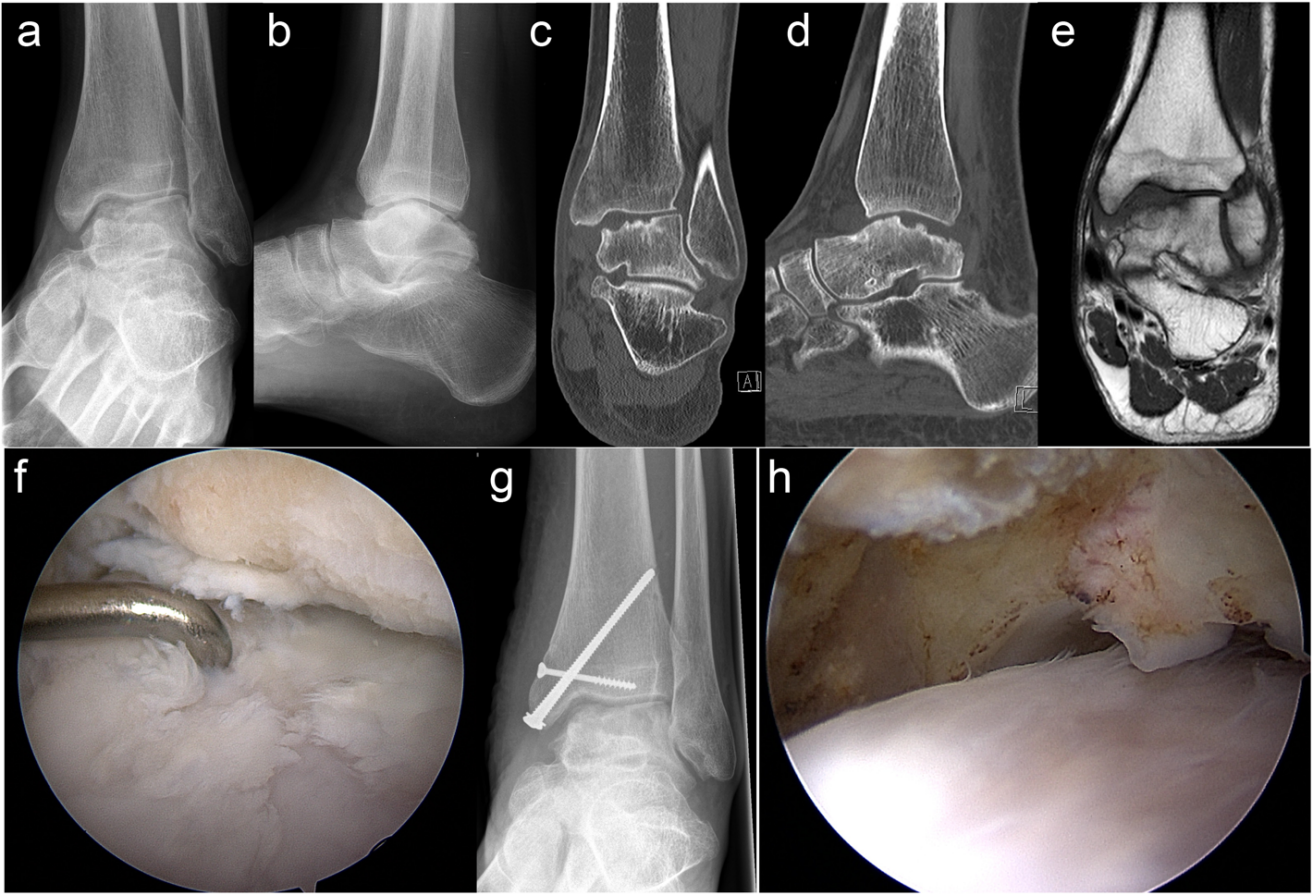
Eighteen-year-old male patient (case 11) with a posttraumatic osteochondral lesion of the medial talus, which was treated with primary combined matrix-associated autologous chondrocyte implantation (MACI) via medial malleolar osteotomy with autologous bone grafting (ABG) from the ipsilateral iliac crest; preoperative antero-posterior (a) and lateral (b) radiographs; preoperative computed tomography scans in the coronary (c) and sagittal (d) plane; preoperative magnetic resonance image in the coronary plane (e); arthroscopic image at the time of chondrocyte harvesting (f) showing the cartilage damage at the medial talus and the status after debridement of a ventral tibial osteophyte; antero-posterior radiograph (g) after MACI/ABG showing screw fixation of the medial malleolar osteotomy; arthroscopic image at the time of hardware removal (h) 23 months after MACI/ABG showing the cartilage surface at the medial talus
